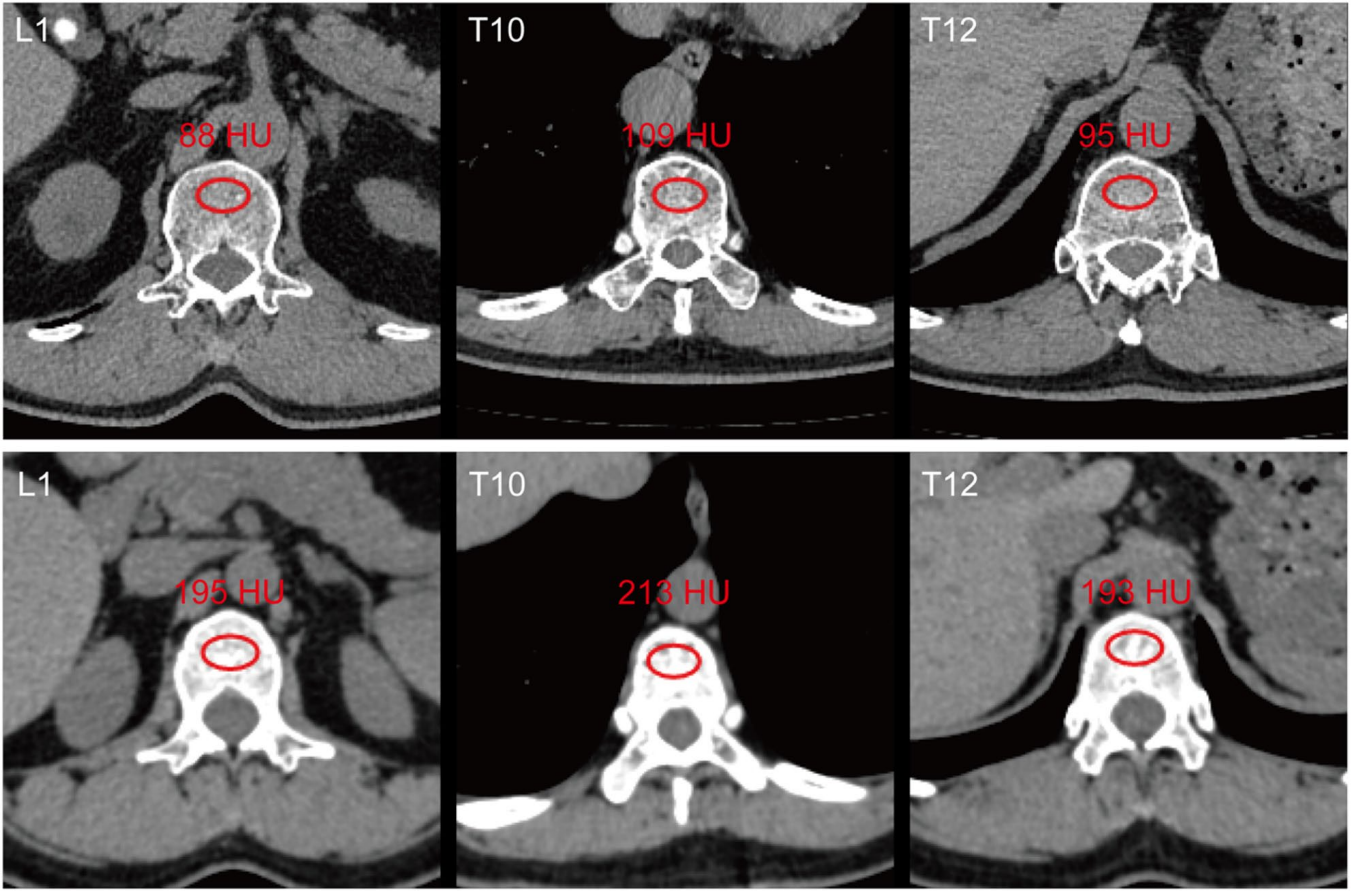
Examples of CT-derived Bone Mineral Density (BMD) Assessment. Representative transverse (axial) CT images at the L1, T10, and T12 vertebral levels showing patients with varying Hounsfield unit (HU) values. The red circular Regions of Interest (ROI) demonstrate the standard placement for trabecular attenuation measurement, positioned in the core of the vertebral body while strictly avoiding the cortical bone and posterior venous plexus. The mean HU value within each ROI is displayed
